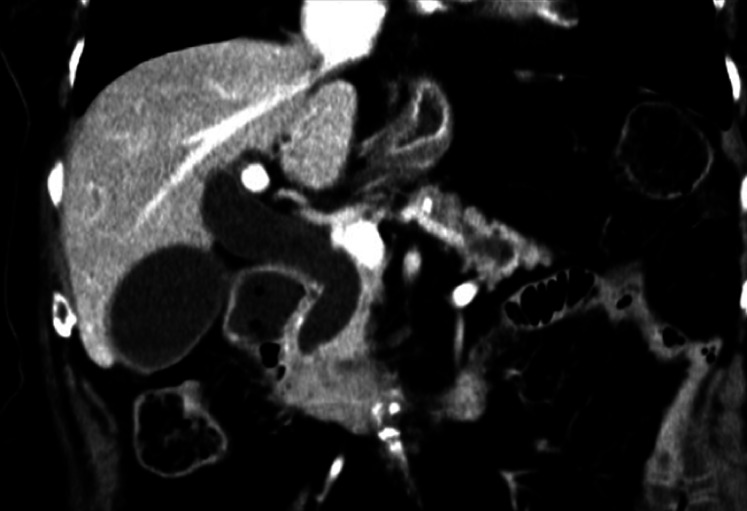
Computed tomography revealed a 35-mm tumor in the pancreatic head, and obstruction of the distal bile duct and duodenum by the tumor was suspected.
